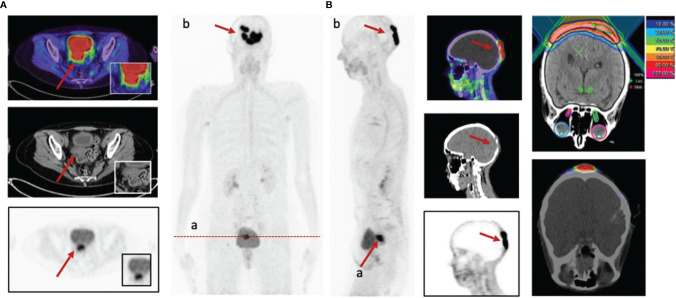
A 58-year-old patient with cervical cancer underwent ^68^Ga-FAPI-PET/CT staging prior to radiotherapy. recurrence of cervical cancer (SUVmax = 14.4) (A). In addition, intracranial metastases showed strong FAPI uptake (SUVmax = 32.3) (B), which allowed for precise delineation of radiotherapy target areas (57).
